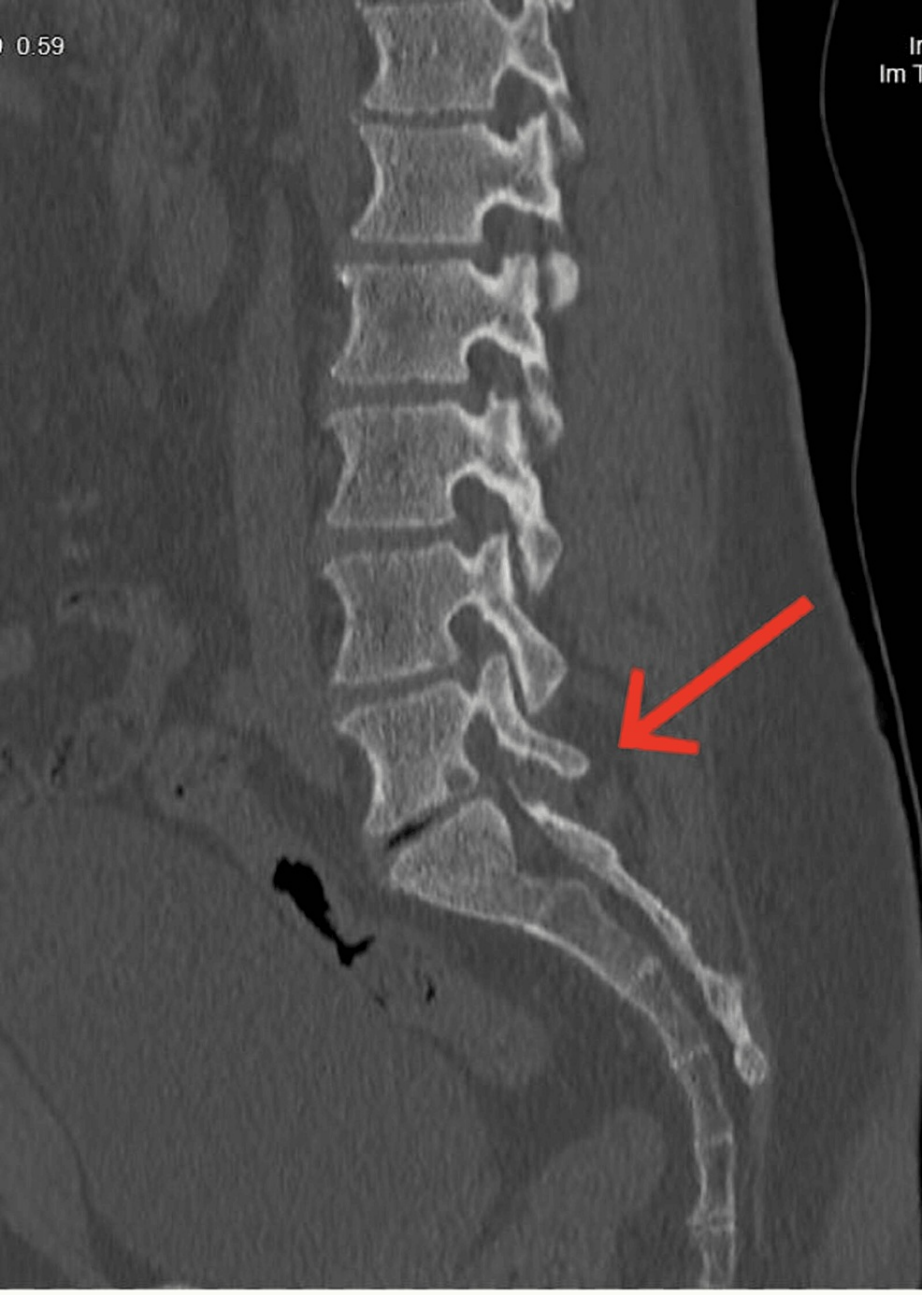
CT scan confirmed the absence of bony osteophytes.
